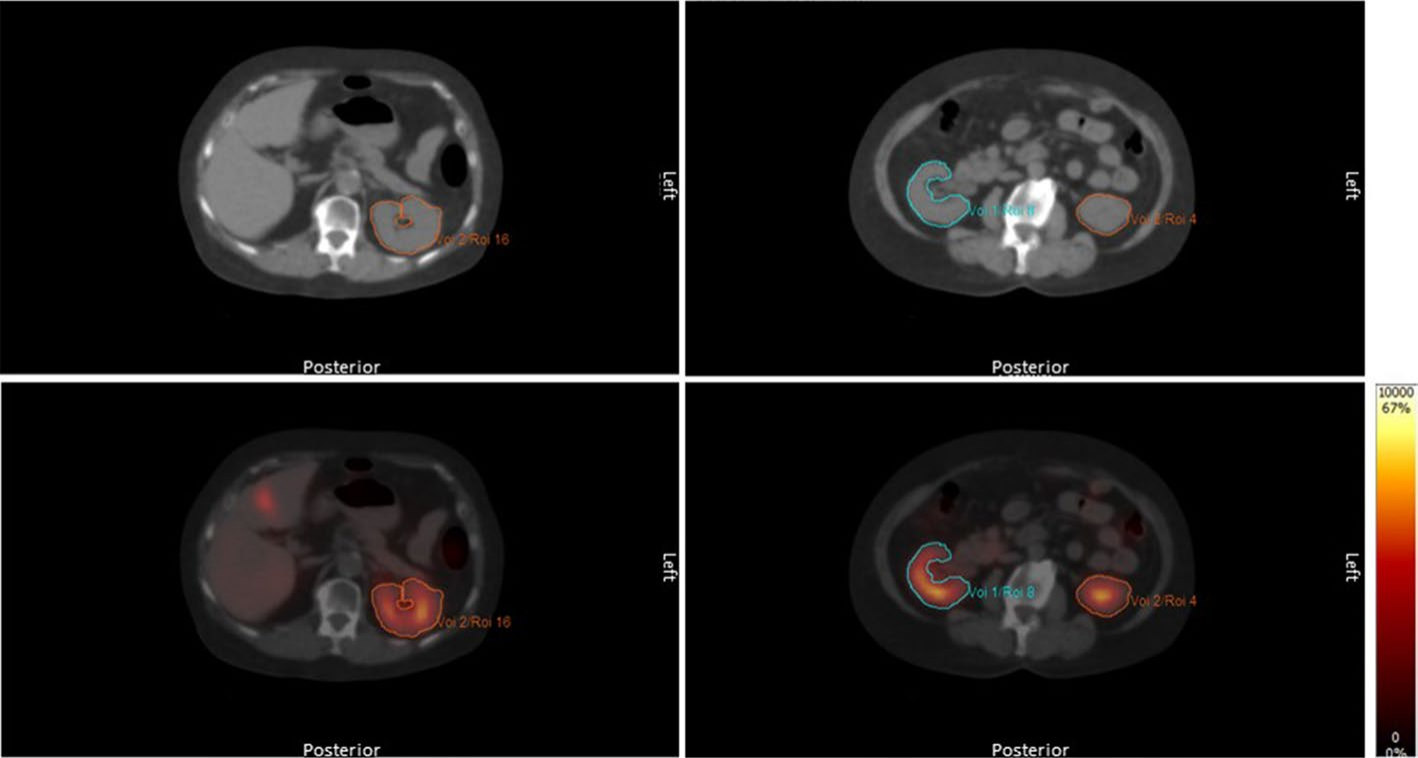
Delineation of kidneys on two transverse slices of a CT scan (top row) and copy to fused images (bottom row) with activity concentration given by the colored scale in units of 100 Bq/cm^3^
